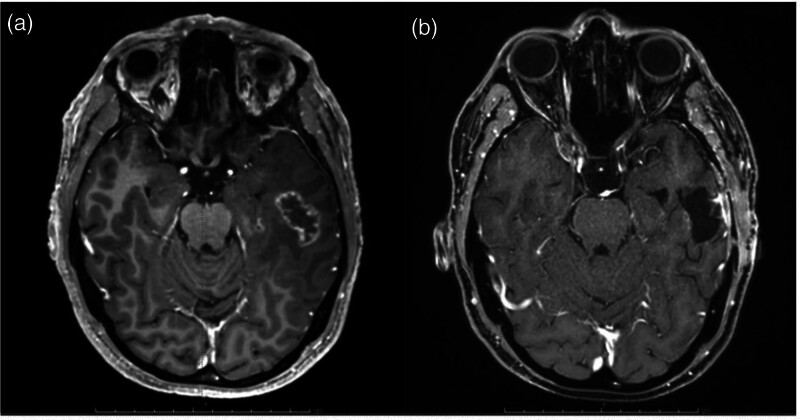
Brain MRI evaluation before (a—November 2021) and after (b—February 2022) the excision of the temporal lesion.
